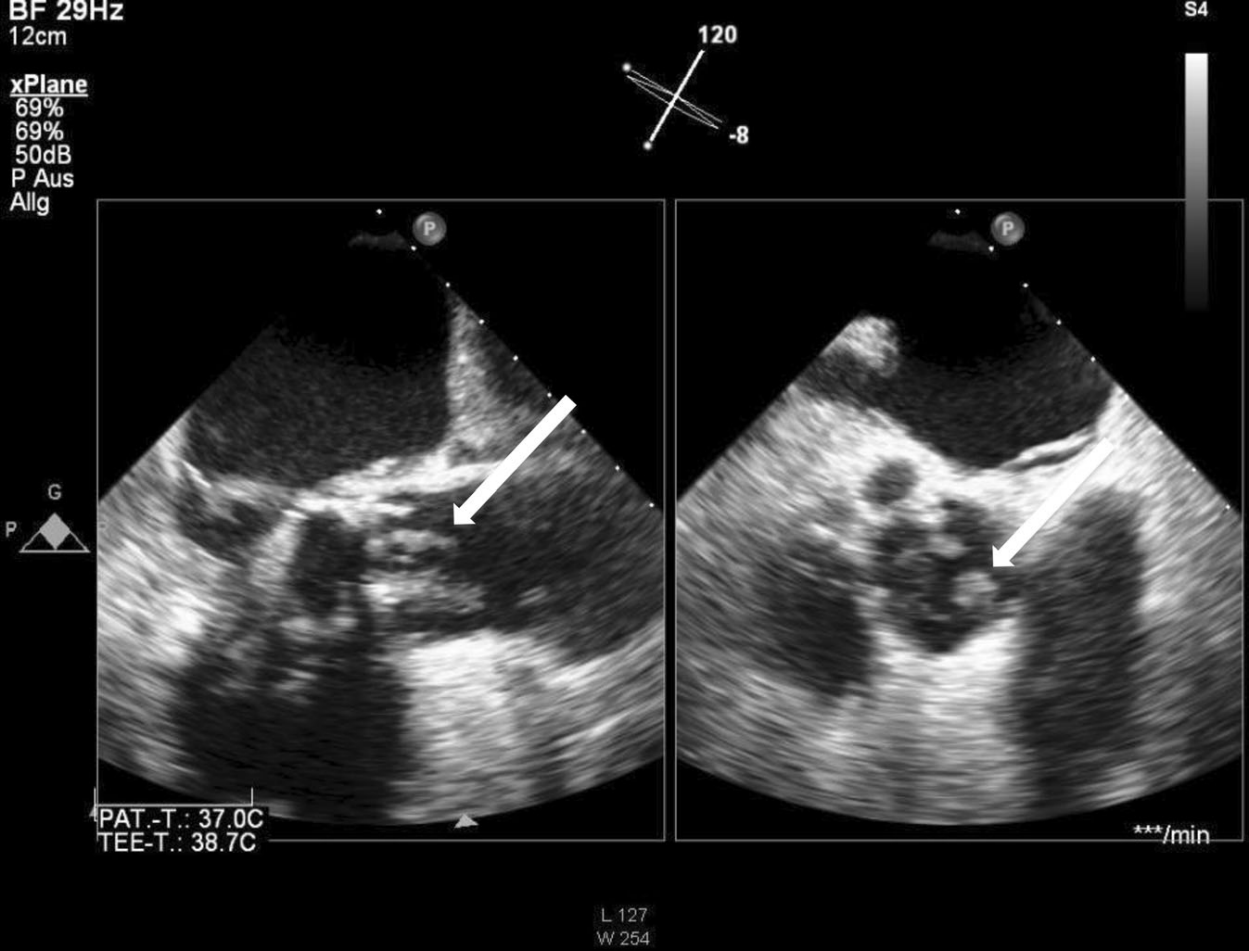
Echocardiography showing vegetations (*arrow*) in endocarditis following aortic valve repair using a bioprosthesis (courtesy of Dr. Frank Heißenhuber, Regensburg)
